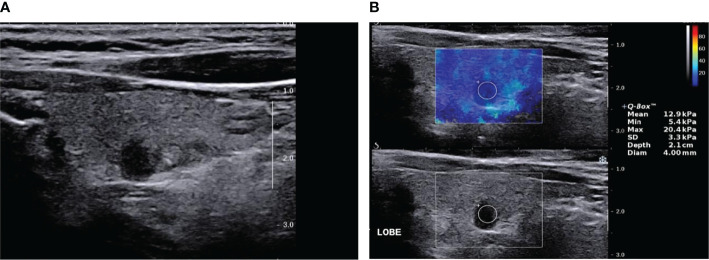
Papillary thyroid carcinoma (SWE false-negative nodule) by two-dimensional ultrasound and elastic ultrasound. (A) A solid, very hypoechoic nodule in the middle-left lobe of the thyroid, 6.0 × 3.0 mm, with an ill-defined margin and taller than wide in shape. The ACR-TIRADS score was 8 and ACR–TIRADS category was 5. (B) The Emax value of the nodule on SWE imaging was 20.4 kPa.
